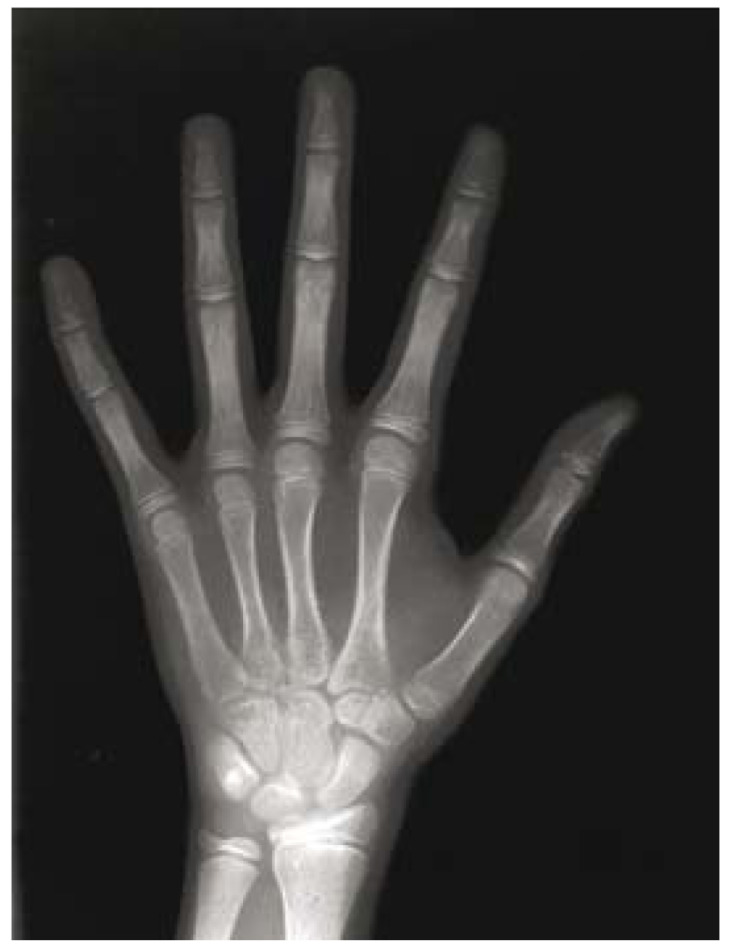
A posteroanterior hand-wrist radiograph from a 10-year-old girl. Equal width of epiphysis and diaphysis of the fifth finger middle phalanx resulted a SMI of 3.
